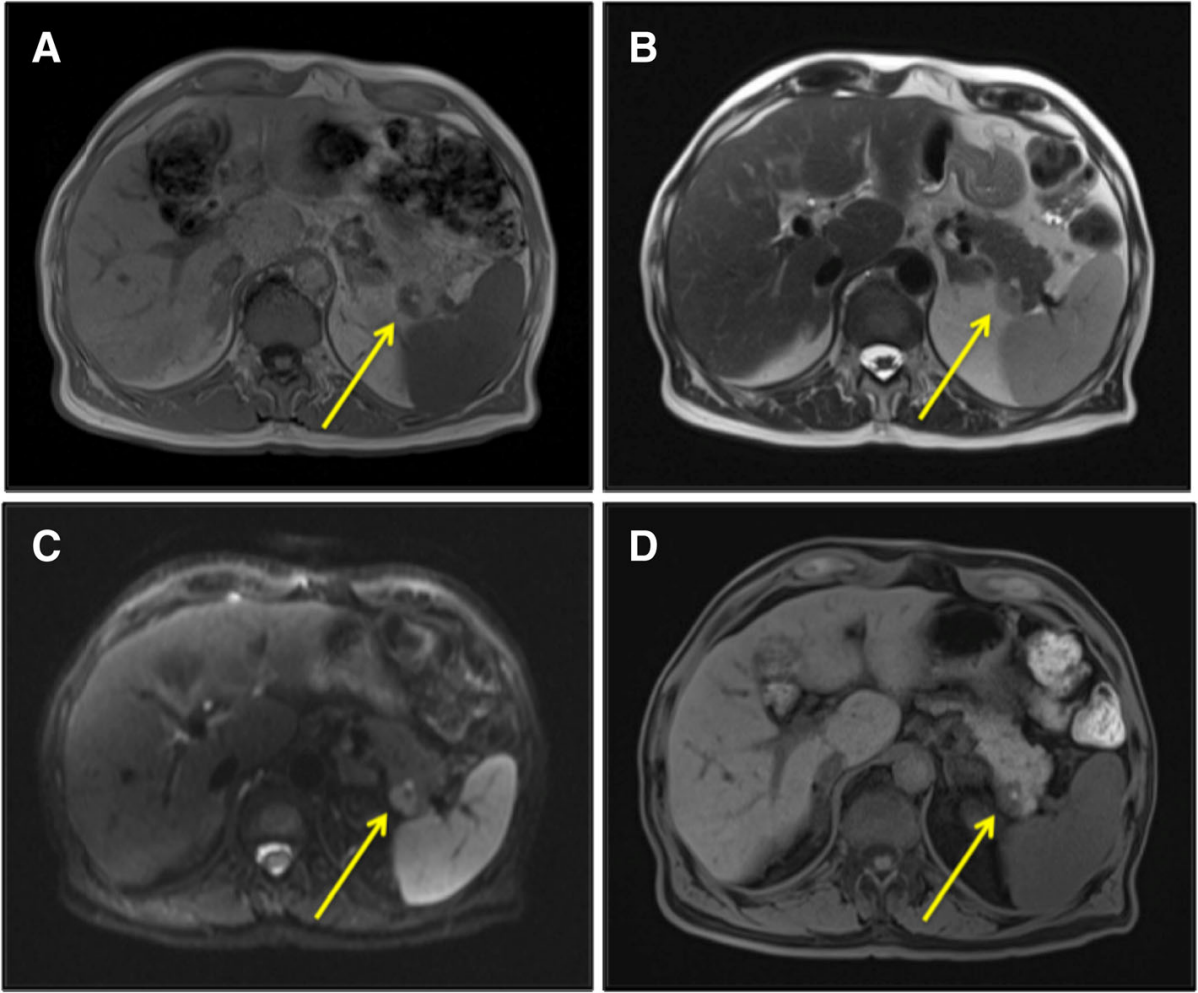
MRI confirmed a 2-cm nodule of the tail of the pancreas hypointense on T1 (a) and hyperintense on T2 (b)-weighted sequence with a focal lesion inside hyperintense on T1. Diffusion-weighted MR imaging shows a high restriction (c) and hypointense in T1 fat sat sequence (d)
